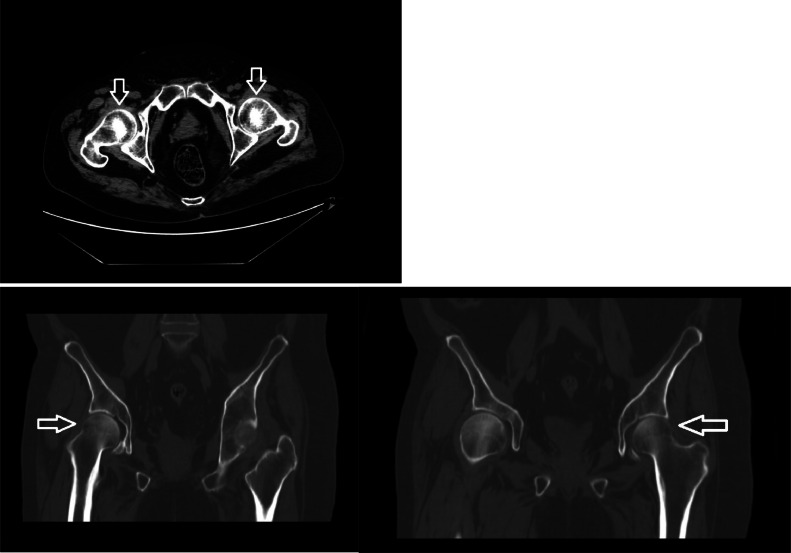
CT of the bony pelvis without contrast notable for mild, increased sclerosis concerning for bilateral, early-stage avascular necrosis of the femoral heads (nonshaded arrows).
